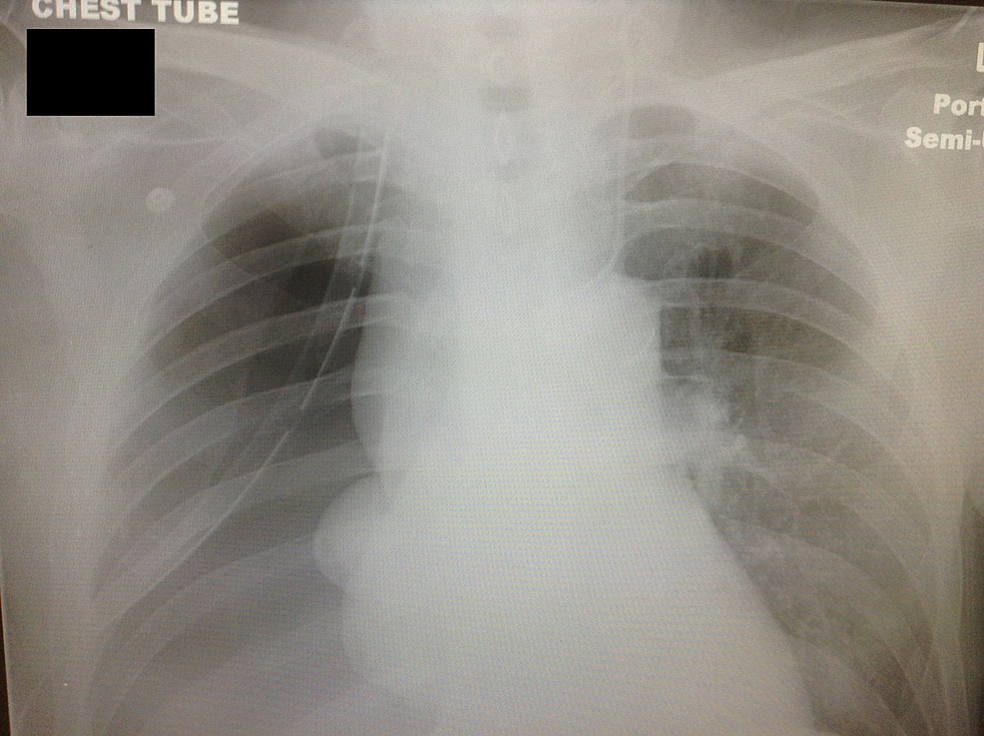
Chest X-ray following thoracostomy tube placement.
